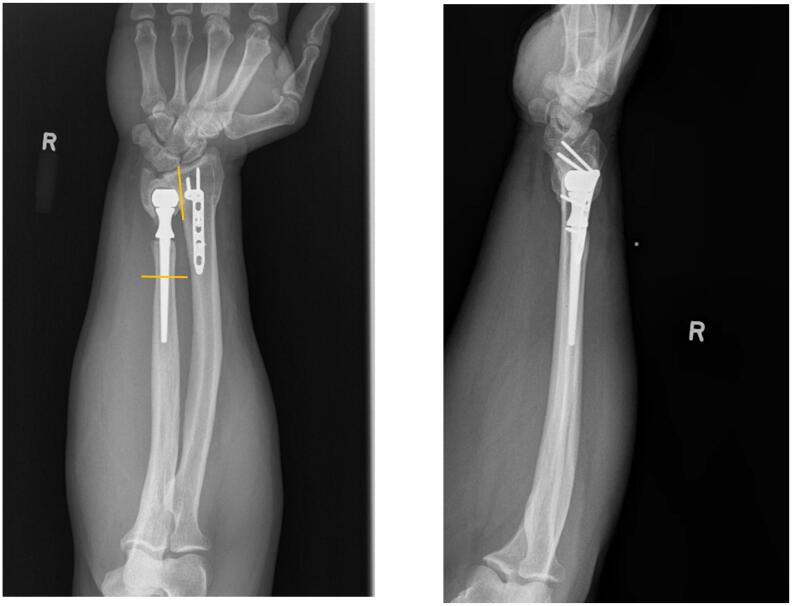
Anteroposterior (AP) and lateral radiographs of the patient's right forearm at presentation, demonstrating the pesudo-arthrosis of the distal ulna to the radius and ulnar stem within the head and diaphysis of the ulna. Some lucency can be seen around the ulnar head but the stem appears well fixed. Lines demonstrating approxiate location of osteotomies can be seen on AP radiograph.
